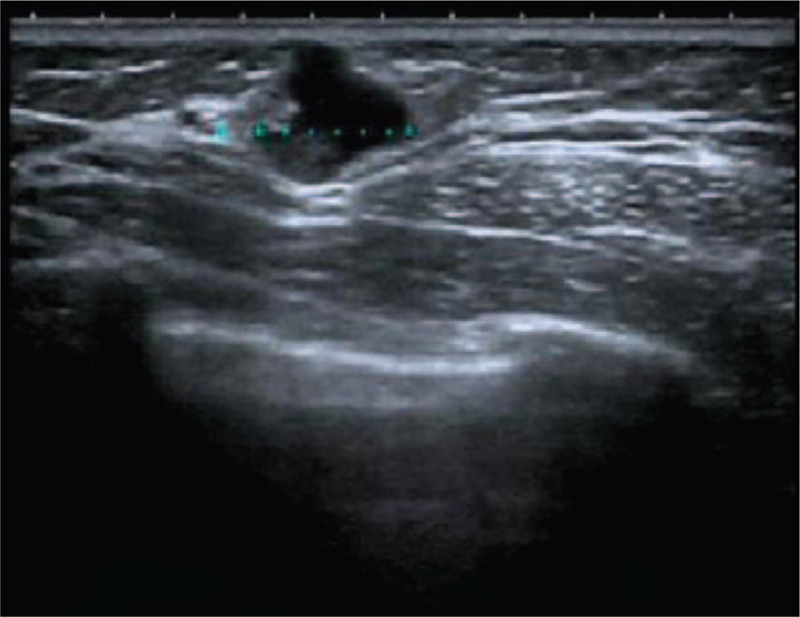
Breast ultrasound revealed a mixed echogenic mass containing cystic and solid components and measured 10.8 mm × 10.1 mm.
